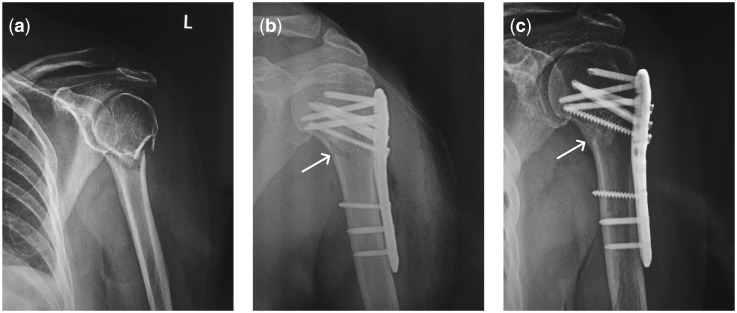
A case of 76 years old female patient diagnosed as comminuted fractures of the left proximal humerus. (a) An X-ray film taken before surgery, in which the comminuted fracture of the surgical neck of humerus could be seen. (b) Radiograph was taken one day after operation for postoperative reexamination. All fractures showed good contraposition and alignment with satisfactory internal fixation. (c) Radiograph was taken three months after operation. Fracture appeared healed with good union. There was neither osteonecrosis nor fracture of humeral head. The internal fixation nails were neither pulled out nor loosened
